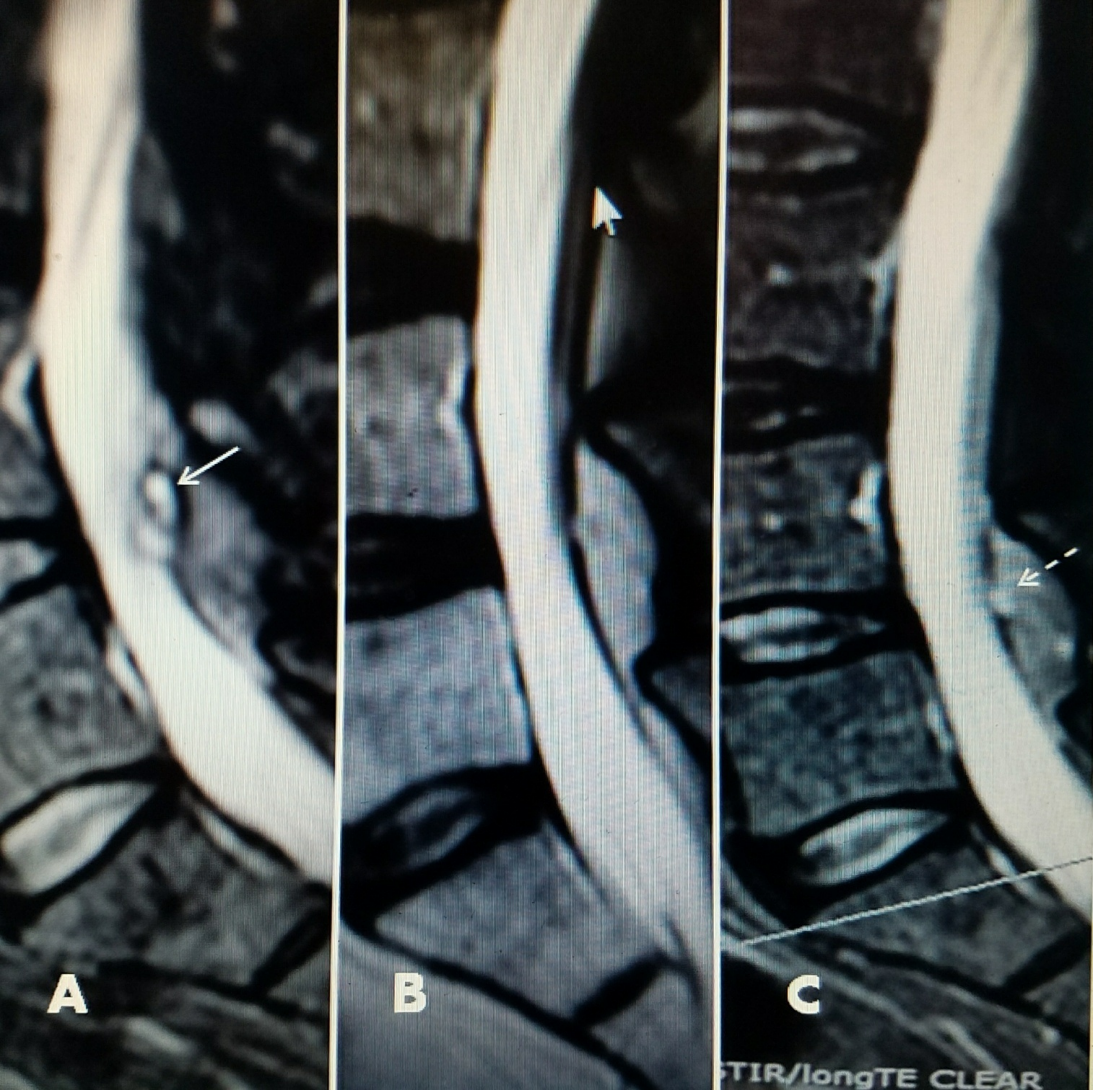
Pre-RF and post-RF followup MRI scans A: Pre-procedure MRI showing 2.2 x 1.4 cm dorsal facet cyst causing lumbar stenosis with neurogenic claudication. Note loculated cystic component (solid white arrow). B: Seven months post drainage and RF ablation of cyst. Patient was asymptomatic.The canal has minimal posterior narrowing. Loculated component disappeared and a smaller dorsal cyst remains. C: Thirty-six months followup MRI. Patient remained asymptomatic. There is microcalcification in cyst (dashed white arrow) but no recurrence of stenosis.
